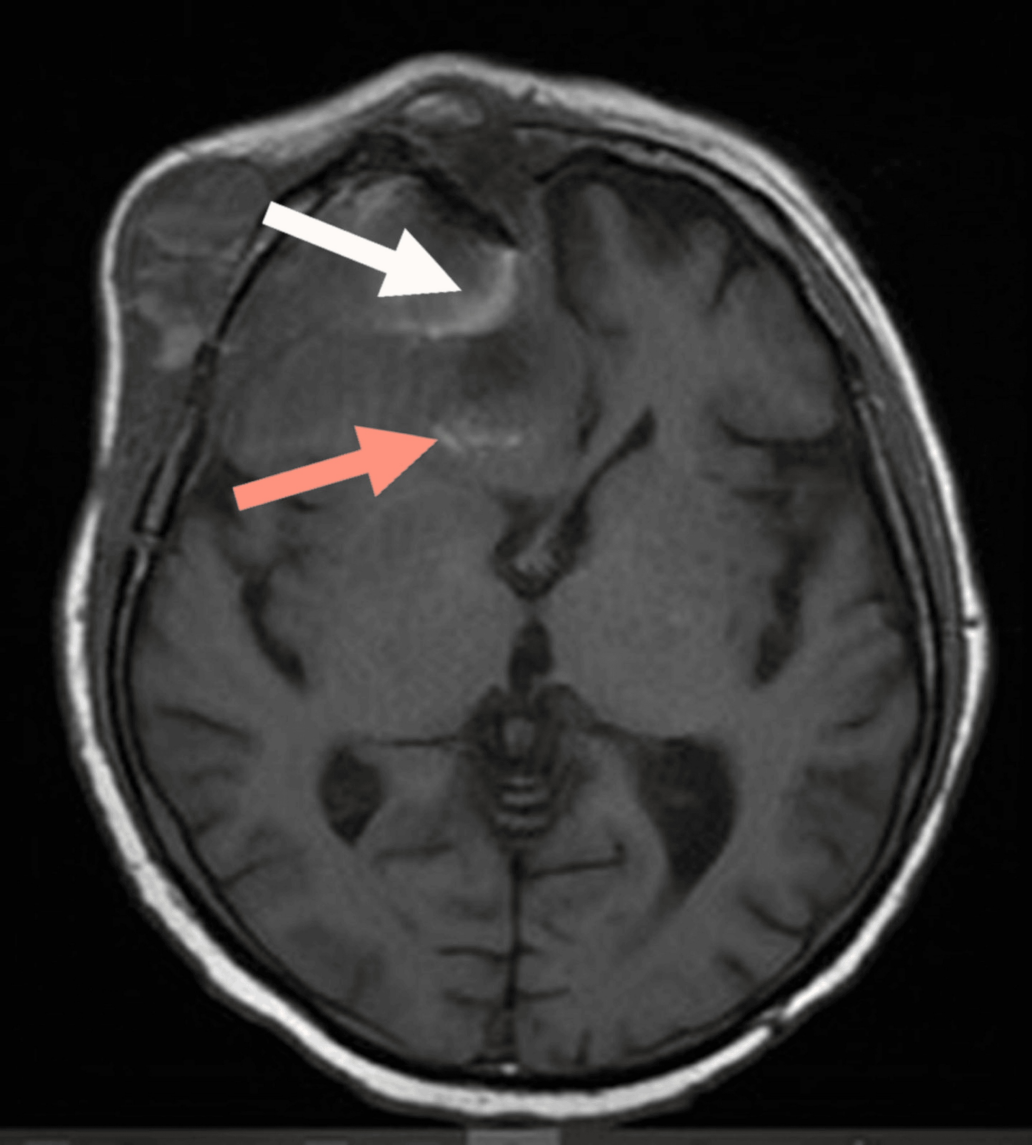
Axial T2-weighted MRI showing bright signal intensity within the mass (white arrow), with significant mass effect on the right lateral ventricle (red arrow).
